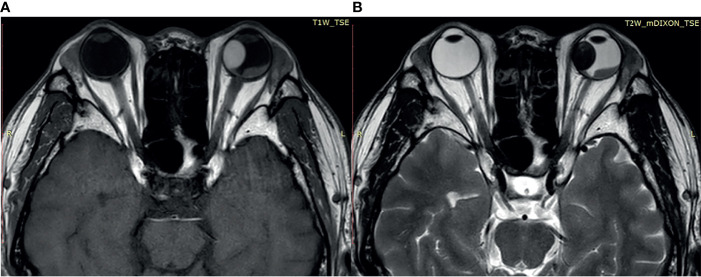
(A) The tumor demonstrates a relatively bright signal on the T1-weighted image. (B) The tumor demonstrates a relatively low signal on the T2-weighted image.
